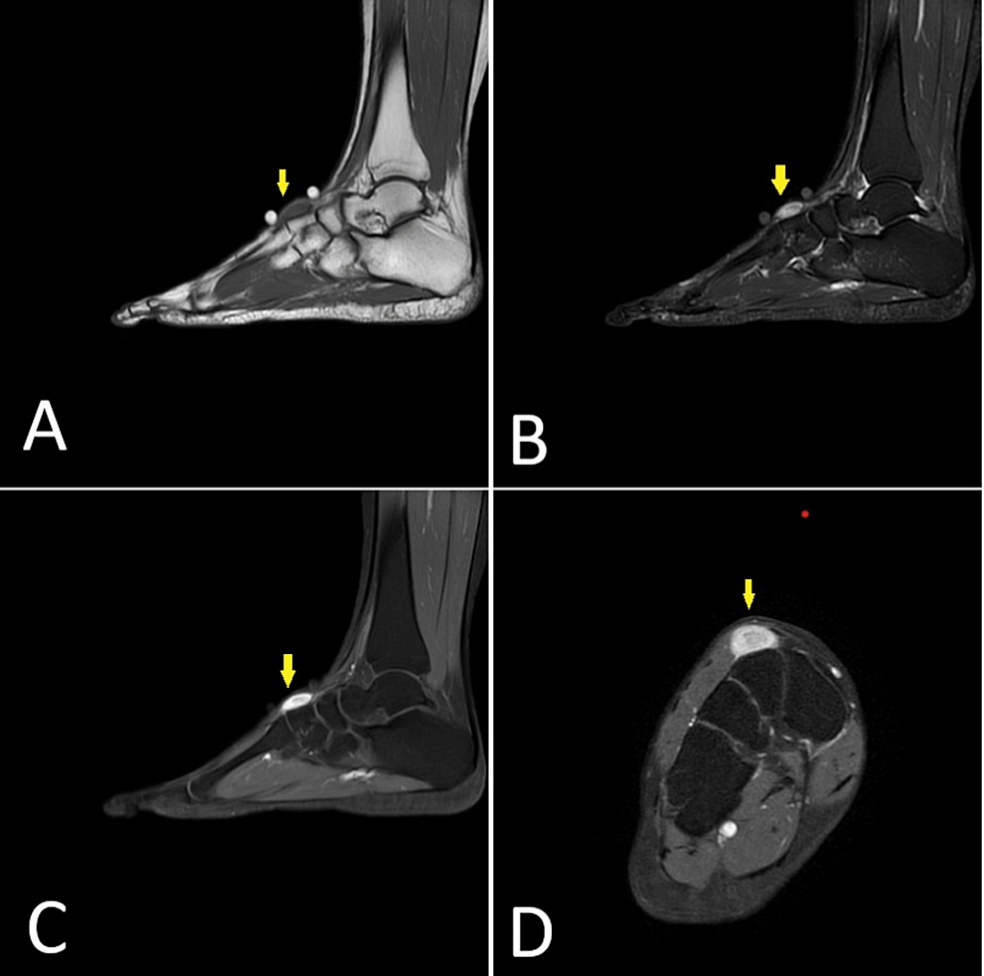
MRI of the foot and ankle showing the lesion on the dorsum of the right foot A: Sagittal T1  FSE showing the lesion (arrow) with a low signal, B: Sagittal FSE STIR where the lesion (arrow) shows a high signal, C: Water–sagittal T1 FSE post Gadolinium with the lesion (arrow) enhances post gadolinium, D: Water–axial T1 FSE post Gadolinium with the lesion (arrow) enhances post gadolinium FSE: Fast spin echo, STIR: Short tau inversion recovery, MRI: Magnetic resonance imaging
